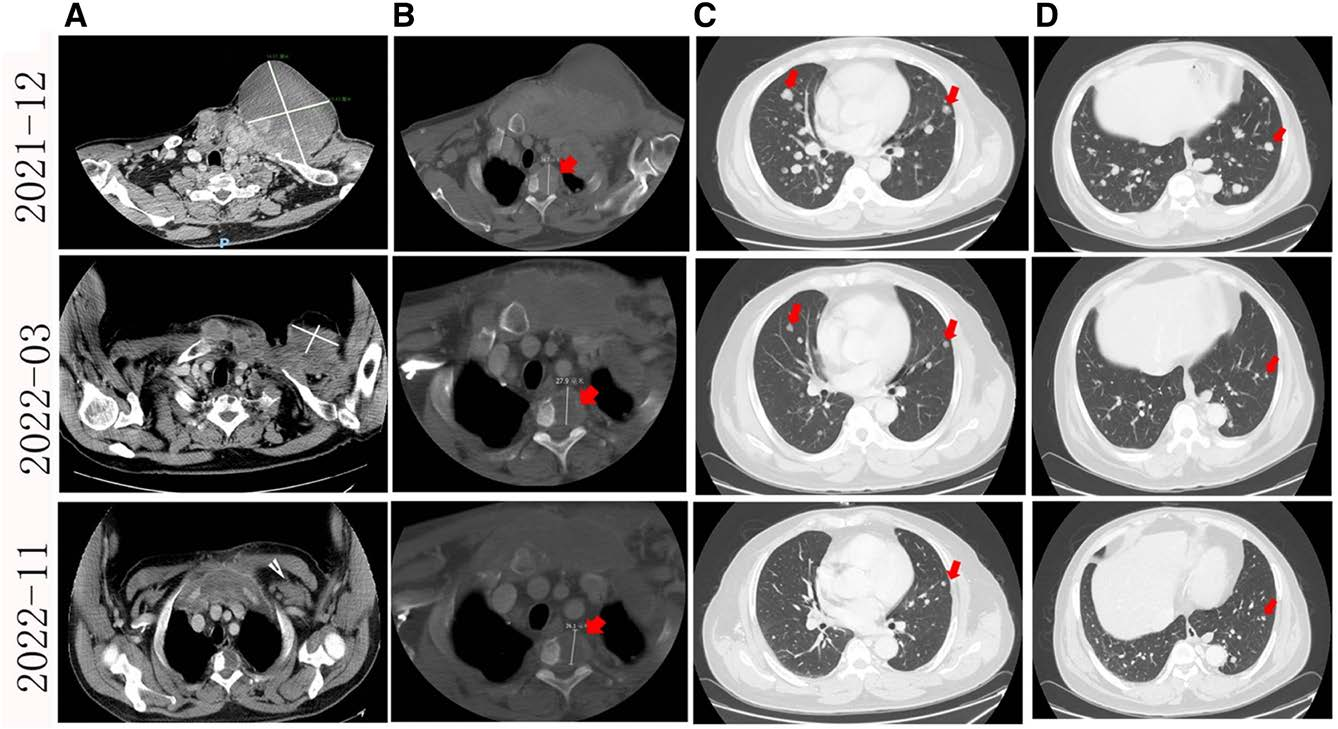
Evaluation results of 2021 to 2012, 2022 to 2003, and 2022 to 2011 CT (plain scan + enhanced scan) (typical lesions are shown as arrows). 2021 to 2012, (A) Left anterior chest tumor, about 14 * 10 cm in size; (B) Spinal bone metastasis; (C–D) Multiple metastatic tumors in both lungs, the larger one is about 2.5 * 1.8 cm. 2022 to 2003, (A) Left anterior chest tumor, about 12.5 * 6.3 cm in size; (B) Spinal bone metastasis; (C–D) Bilateral lung metastasis, the larger one is about 1.8 * 1.4 cm. 2022 to 2011, (A) Left anterior chest tumor, about 11.1 * 4.2 cm in size; (B) Spinal bone metastasis. (C–D) Bilateral lung metastasis, the larger one is about 1.7 * 1.2 cm.
